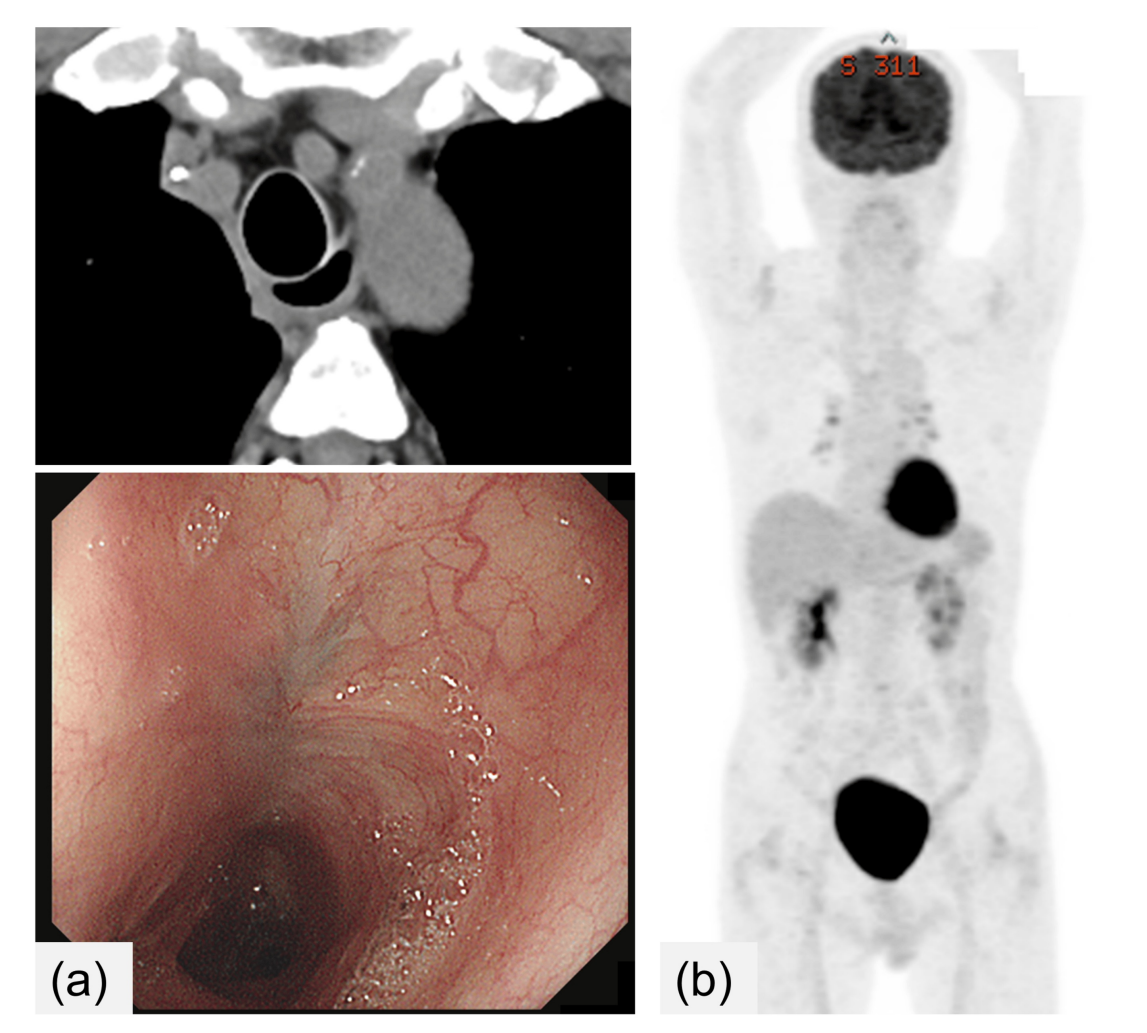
Images after chemotherapy (a) CT and endoscopy findings after four chemotherapy cycles showed fistula closure and complete remission of DLBCL. (b) Positron emission tomography (PET) image after six chemotherapy cycles indicated complete metabolic remission.
